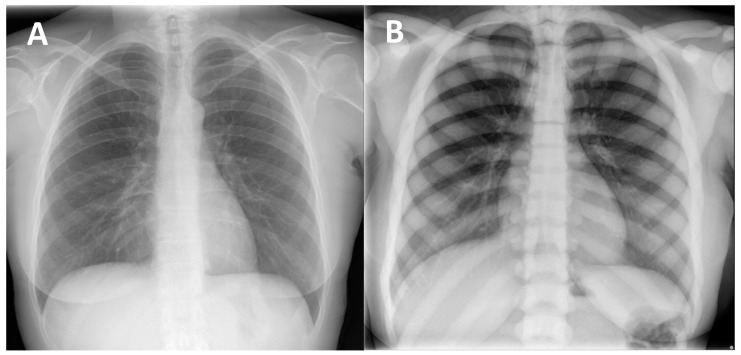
(A) conventional radiography of the chest of a young healthy female (shown as comparison). (B) conventional radiography of the chest in a 29-year-old female diagnosed with osteopetrosis (clinical and radiological diagnosis in absence of a pathogenic variant in osteopetrosis-related genes). All the skeletal segments included are affected by diffuse and markedly increased bone density (spine, ribs, clavicle, scapulae, humerus): ‘Marble bone’ appearance.
